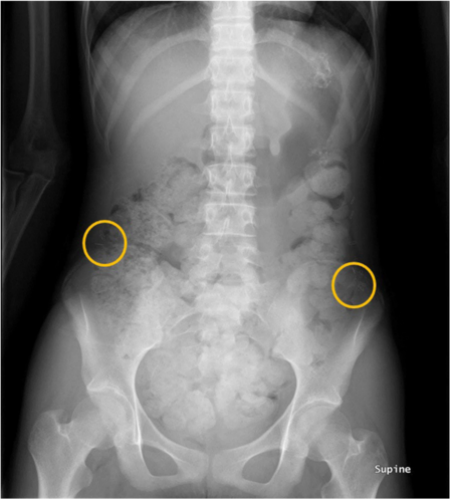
Postoperative roentgenogram showing the position of metallic clips applied to each ovary
